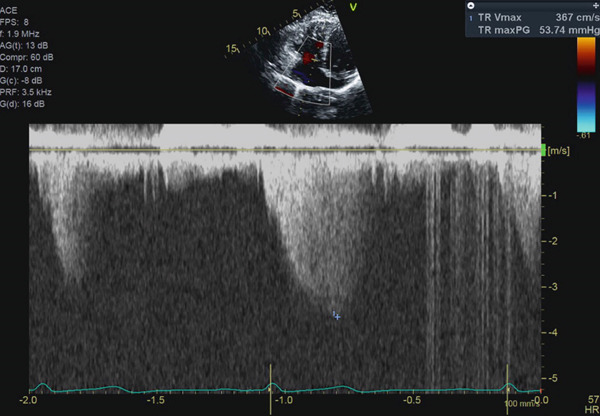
Transthoracic echocardiogram. Apical four‐chamber view. Continuous wave Doppler estimating an elevated tricuspid regurgitation peak gradient (TRPG) at 54 mmHg, suggestive of increased pulmonary artery pressure.
